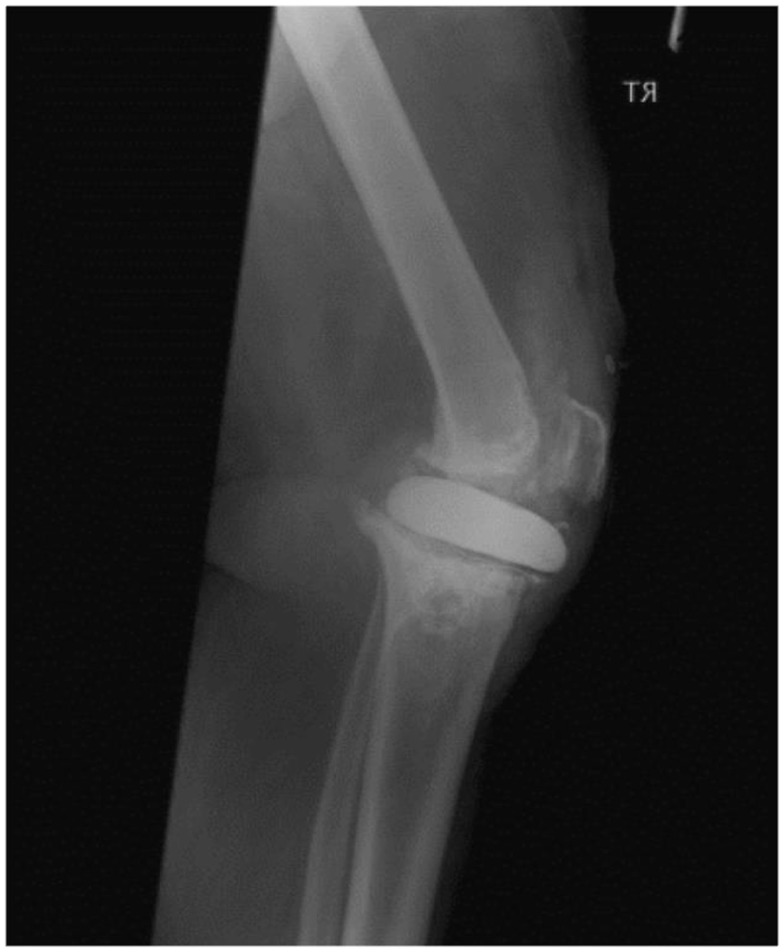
Partial rupture of the patella tendon in Case 3 demonstrated by the lateral radiographs of the knee showing anterior displacement of the static cement spacer blocks. Note that the block was not keyed in.
